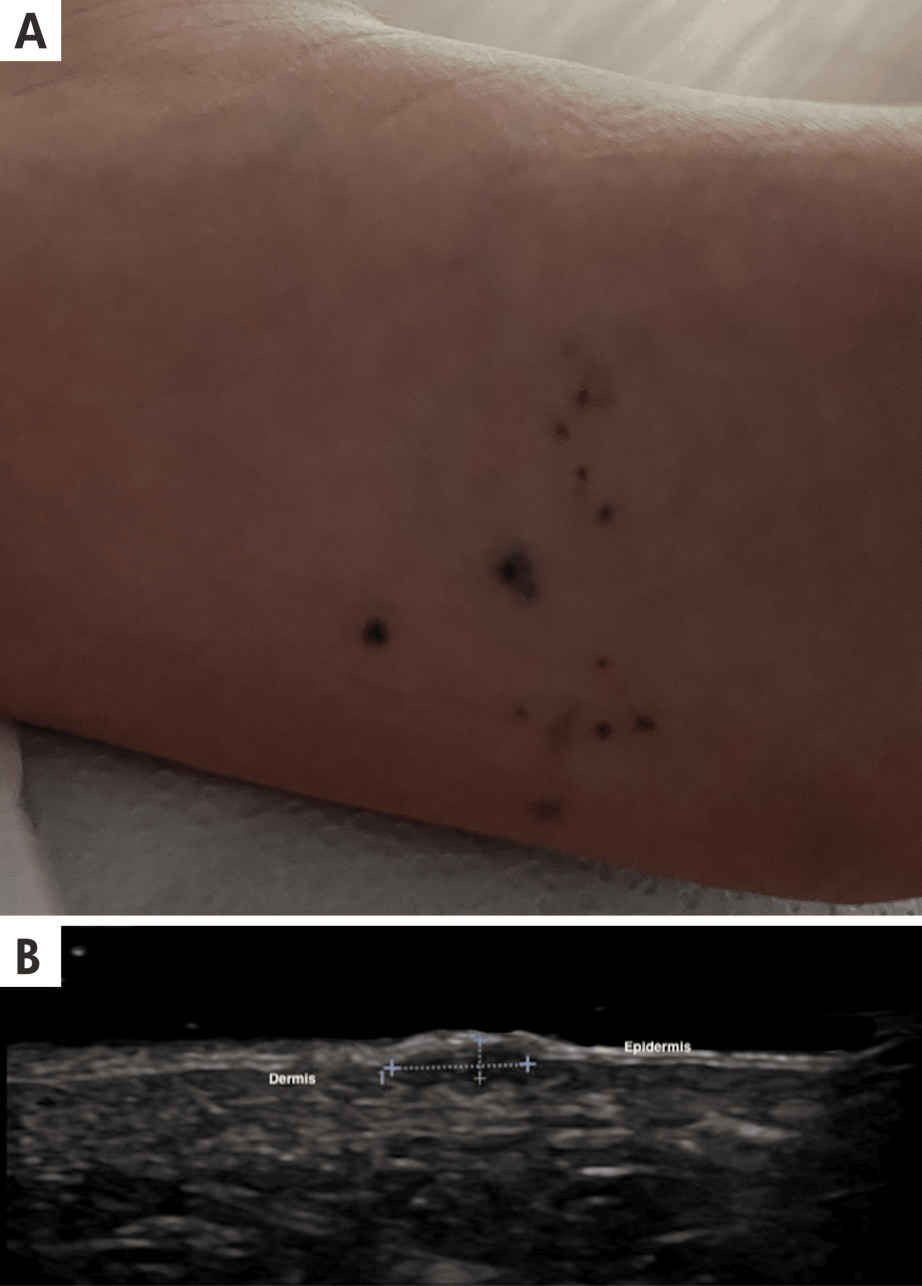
Dermatological ultrasound and clinical imaging of cherry angioma A: A 6-year-old male patient had maculopapular, cup-shaped, red-wine-colored lesions ranging from 1 mm to 4 mm, randomly distributed but grouped in the left plantar arch region. B: The longitudinal ultrasound view and grayscale ultrasound of the foot show between calipers (+) a small hypoechoic, exophytic nodule, causing upward displacement of the epidermis. This epidermis has the classic hyperechoic bilaminar appearance in the sole.
